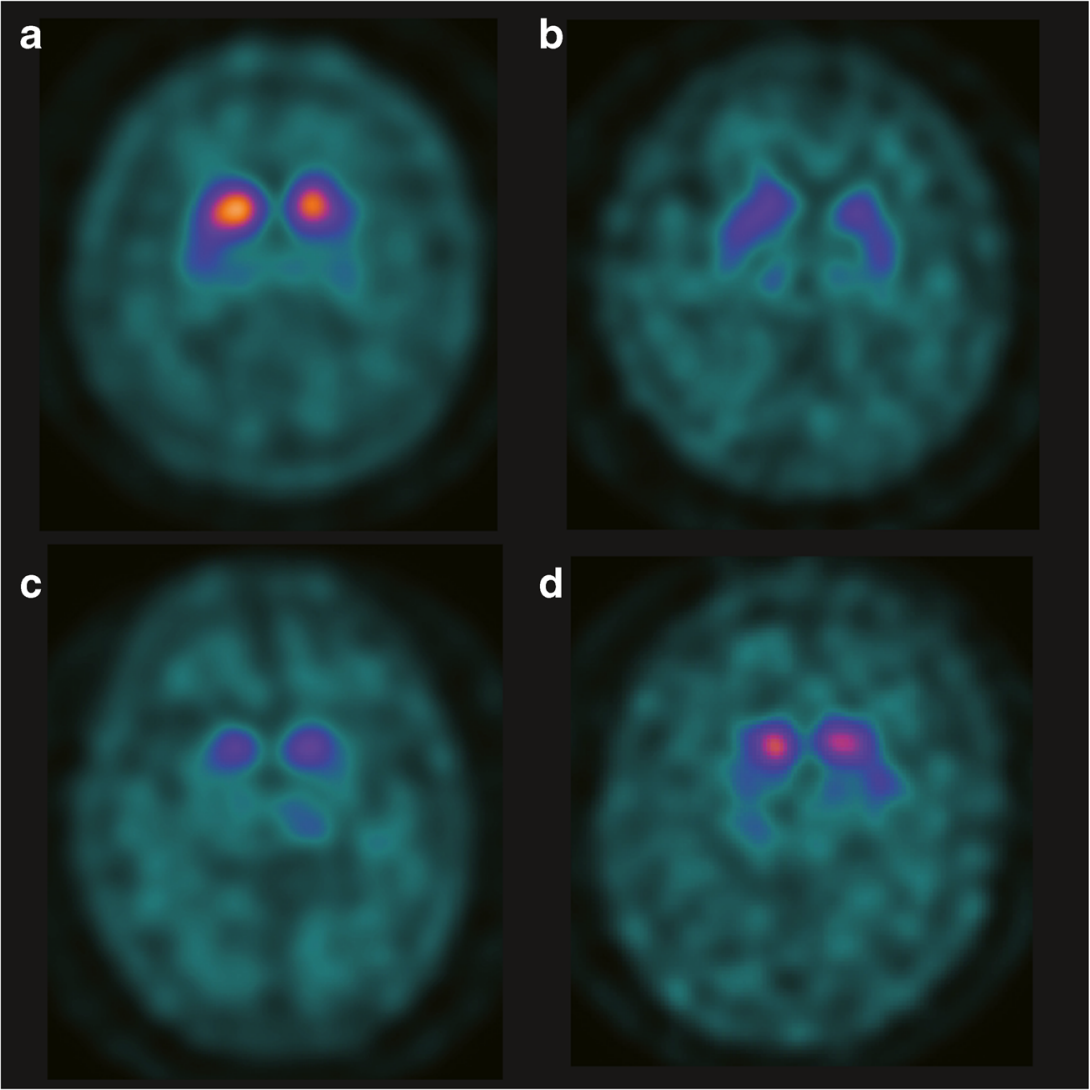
DAT SPECT images of representative cases of PD, DLB, PSP, and MSA. a A representative case of PD (aged 64 years, Female). b A representative case of DLB (aged 79 years, Female). c A representative case of PSP (aged 86 years, Female). d A representative case of MSA (aged 56 years, Male). Abbreviations: *DLB* dementia with Lewy body, *MSA* multiple system atrophy, *PD* Parkinson’s disease, *PSP* progressive supranuclear palsy
